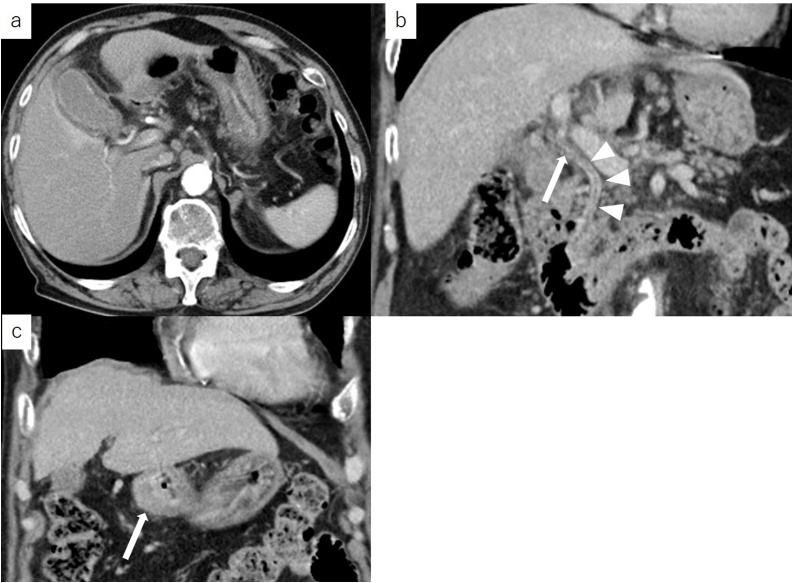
Contrast-enhanced computed tomography findings. a. Swelling and thickening of the gallbladder wall; concentration of fat in the surrounding tissue was also observed to have increased. b. There were no stones in either the cystic duct (arrow) or the extrahepatic bile ducts (arrowhead). c. Thickening of the gastric wall at the pylorus was suspected (arrow).
